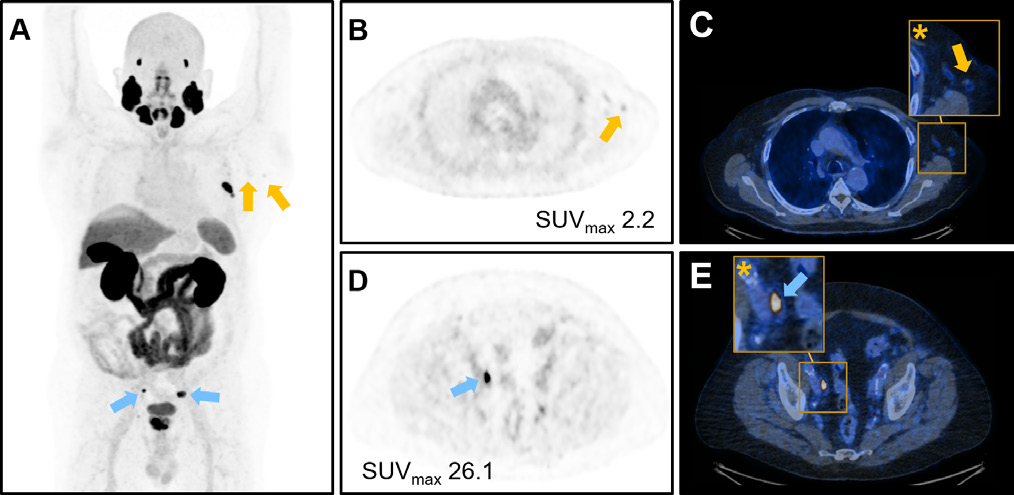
Representative images of a 69-year-old patient who underwent [^18^F]-PSMA-1007 PET/CT for staging of newly diagnosed prostate cancer with mildly [^18^F]-PSMA avid axillary lymphadenopathy (orange arrows) after second COVID-19 vaccination on the left side-with Pfizer-BioNTech. (A) Maximum intensity projection image showing [^18^F]-PSMA avid left axillary lymph nodes (orange arrows) as well as [^18^F]-PSMA avid pelvic lymph node metastases (blue arrows), (B) axial PET image of the axilla and (C) corresponding fused PET/CT images with magnified image of the left axilla (asterisk), (D) axial PET image of lymphe node metastases in the pelvic region and (E) corresponding fused PET/CT images with magnified image of the left pelvis (asterisk). PET, positron emission tomography; PSMA, prostate-specific membrance antigen; SUV_max_, maximum standardized uptake value.
